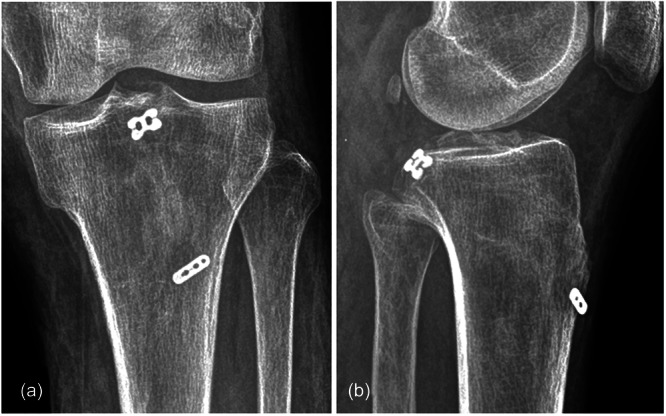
Post‐operative plain radiographs showing fracture union with adjustable‐loop suspensory device. (a) Anteroposterior and (b) lateral views of the knee are shown.
